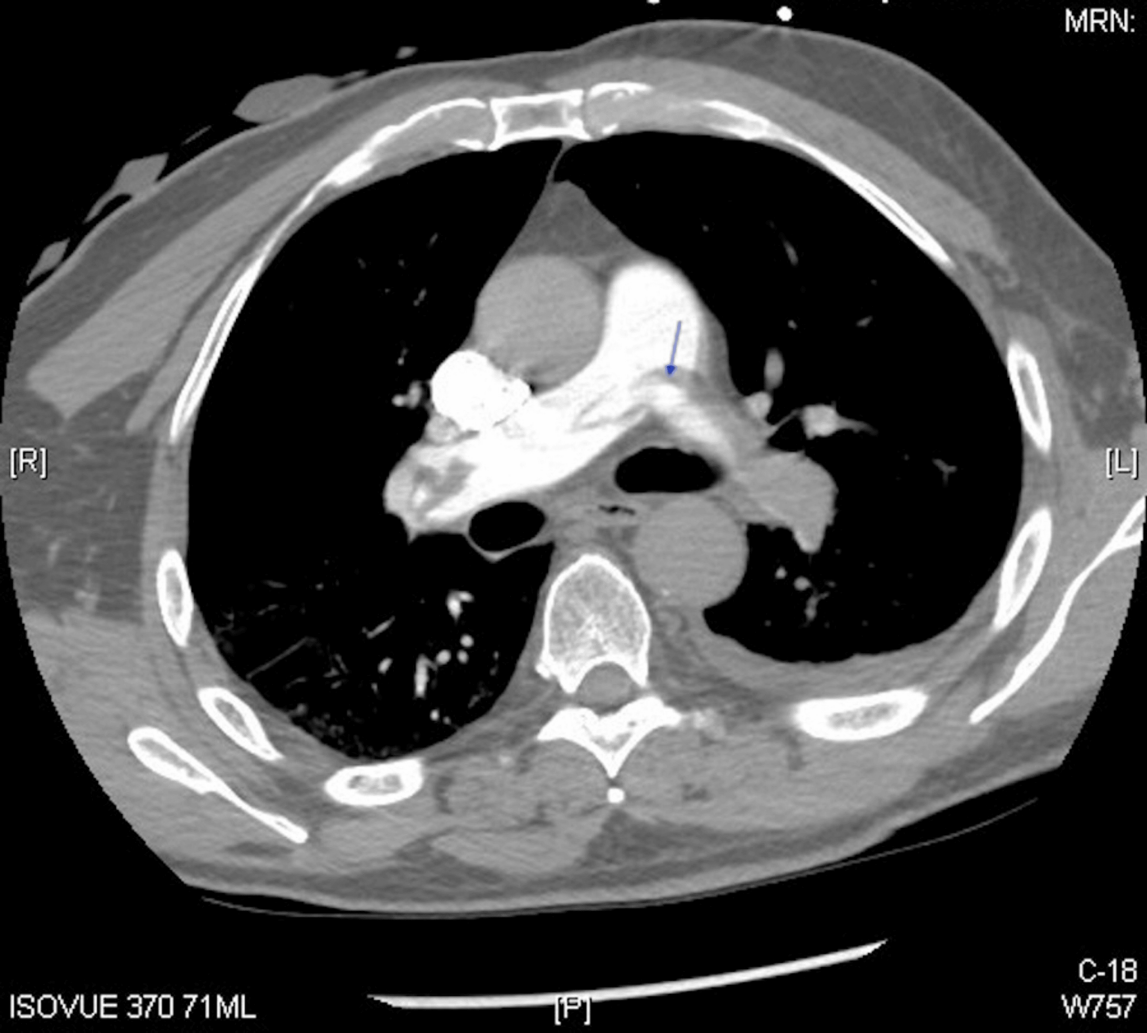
Computed tomography angiography (CTA) demonstrating a saddle pulmonary embolus (arrow)
